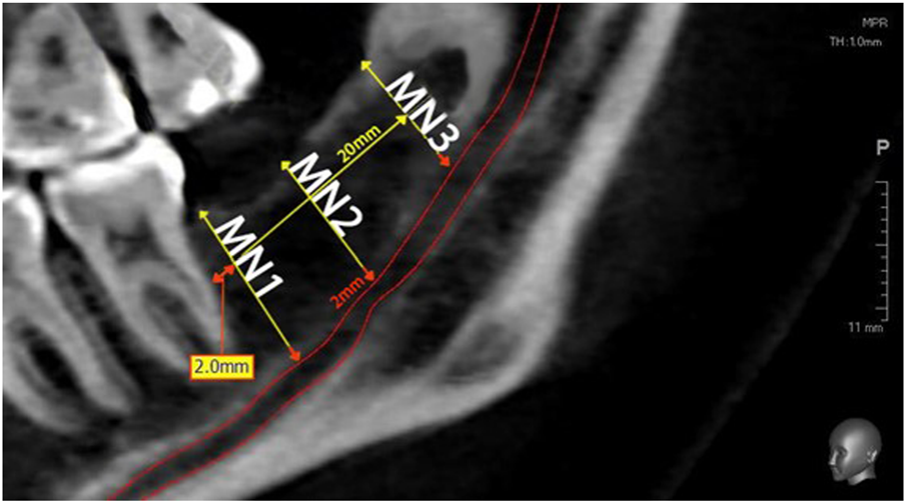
Sagittal section of retromolar region in cone‐beam computed tomogram image with mandibular canal marked in red and measuring points marked in yellow
